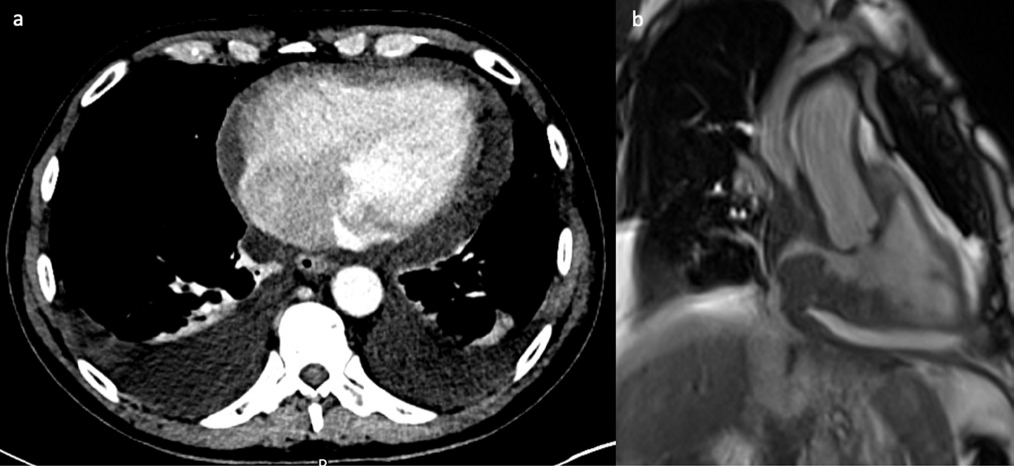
(a) Contrast-enhanced CT showing small a pericardial
